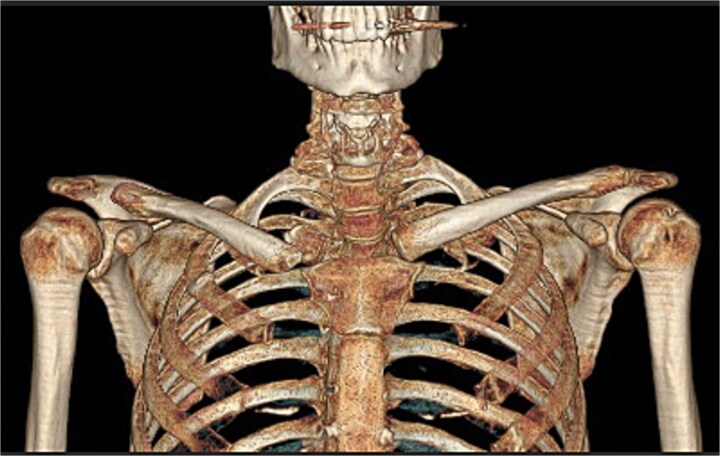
Pre-operative CT imaging showed signs of pseudoarthrosis involving the sternoclavicular joint.
